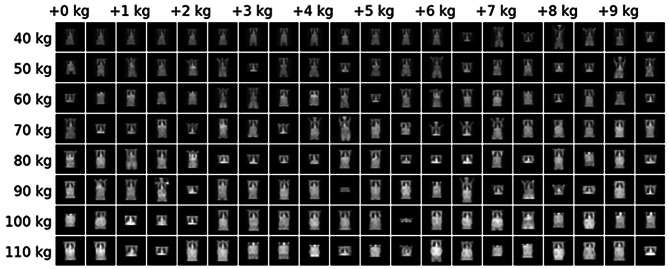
Selected CT scout images from lower to higher weights in kg. Input images are shown as they are supplied to the deep network during training. A large diversity of shapes is visible, with images with larger body weight having larger shapes and more attenuation
